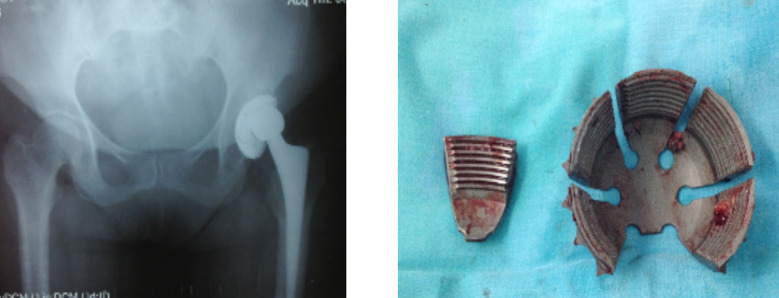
(a) Implant radiograph and photo of a 53-year-old female patient. The patient underwent revision surgery with the diagnosis of acetabular cup breakage in the 12^th^ year of THA. (b) The broken wing of the acetabular cup during revision surgery.
